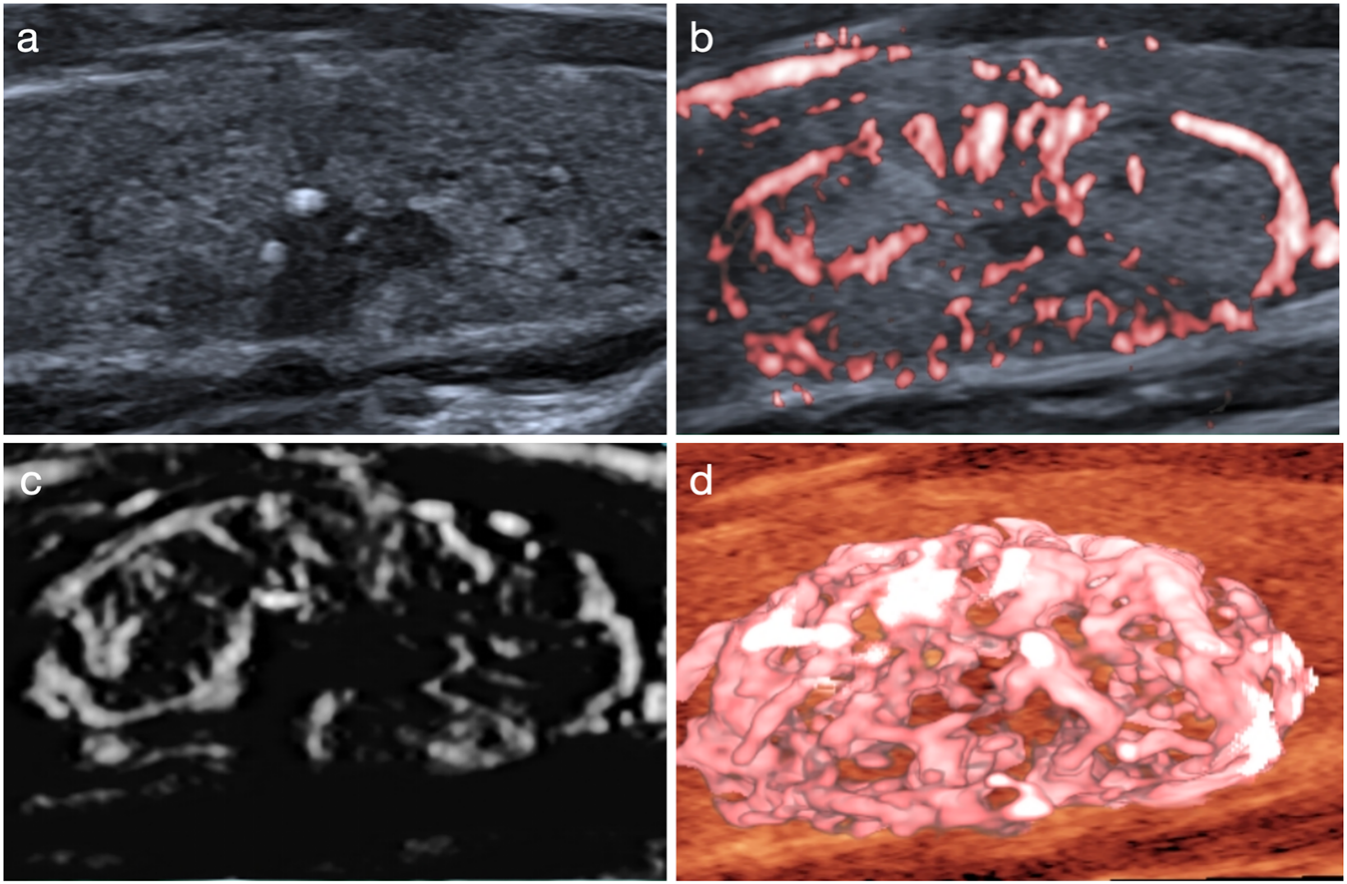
Ultrasound images of a solid isoechoic nodule of 2.0 cm with punctate echogenic foci in the right thyroid lobe of a 60-year-old female patient. After adjustment by the wheel-like microflow pattern on SMI, one-level downgrade was made for ACR TI-RADS, ATA RSS, KSThR TIRADS, EU-TIRADS and C-TIRADS, and the biopsy recommendations of ACR TI-RADS and KSThR TIRADS were exempted. Cytopathological result showed a benign nodule of Bethesda II. a Greyscale ultrasound, b CSMI, c MSMI, d Smart-3D
